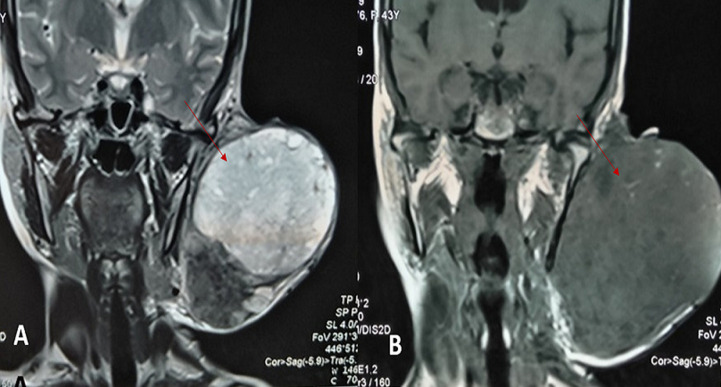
parotid MRI in axial sections showing a left parotid mass in T2 hypersignal A) and T1 hyposignal B)
